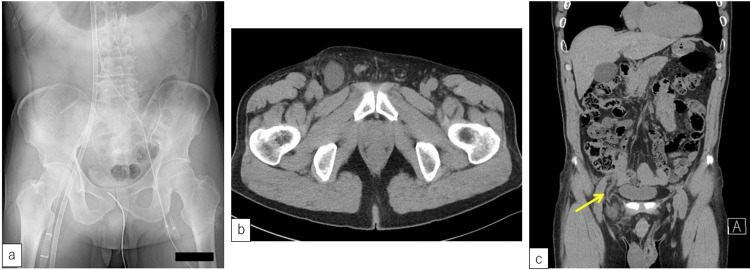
Abdominal X-ray during VA-ECMO and abdominal computed tomography of the femoral hernia involving the appendix. a: VA-ECMO blood inflow cannula is inserted through the right femoral artery, and the blood outflow cannula is inserted through the right femoral vein, and an intra-aortic balloon pumping catheter is inserted through the left femoral artery; b: despite the presence of fluid retention, no signs of perforation are observed; c: the appendix is observed in the right femoral hernia (arrow). (b: axial section; c: coronal section). VA-ECMO: veno-arterial extracorporeal membrane oxygenation
